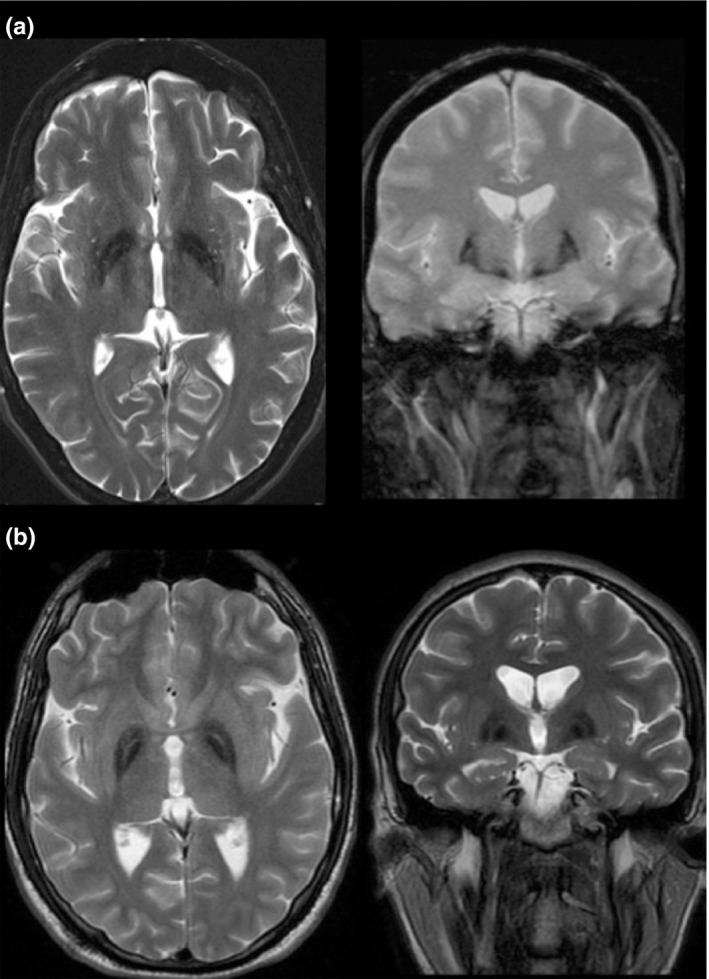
MRI findings in dominant MPAN. T2‐weighted imaging of brain at the level of the globus pallidus (axial left; coronal right) showing hypointense signal indicative of iron accumulation in subject 18‐411 (panel a, presumed dominant case) and subject 248 (panel b, recessive case). Characteristic of MPAN and seen in both cases is the preservation of isointense signal in the medial medullary lamina, which localizes between the globus pallidus interna and externa. MPAN, membrane protein‐associated neurodegeneration; MRI, Magnetic resonance imaging
